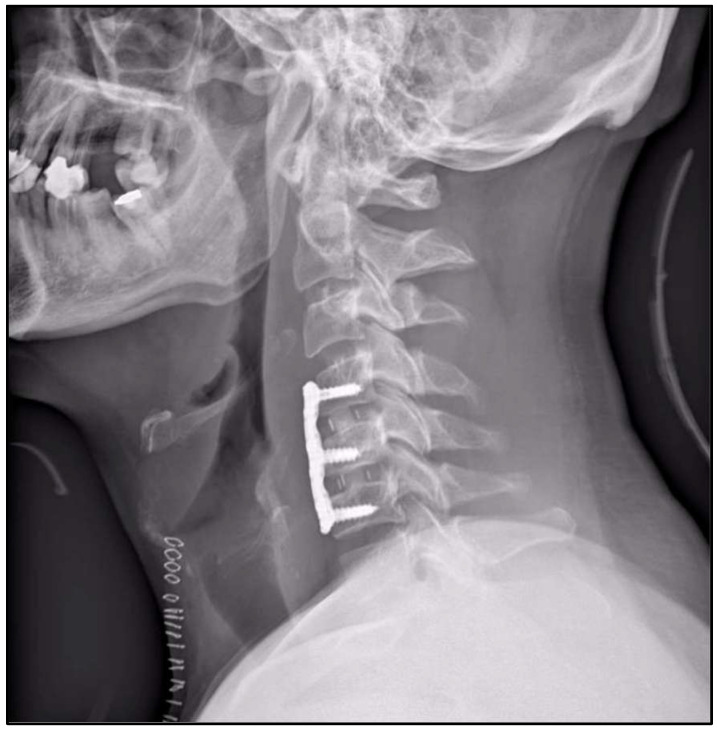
Postoperative lateral cervical radiograph demonstrating ACDF at C4–C5 and C5–C6 with interbody cages and an anterior plate spanning C4–C6.
